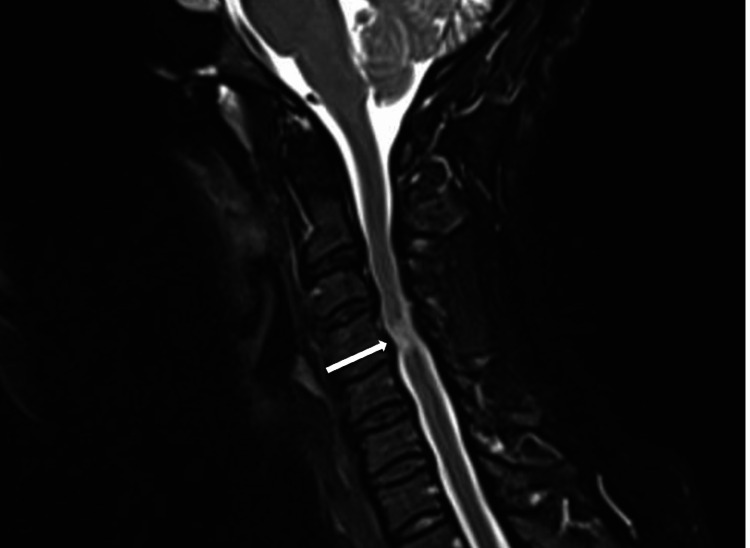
Sagittal T2-weighted MRI of the cervical spine with neutral neck position showing cord signal abnormality at C4-C5 with cord atrophy at that level without obvious extrinsic cord compression.
